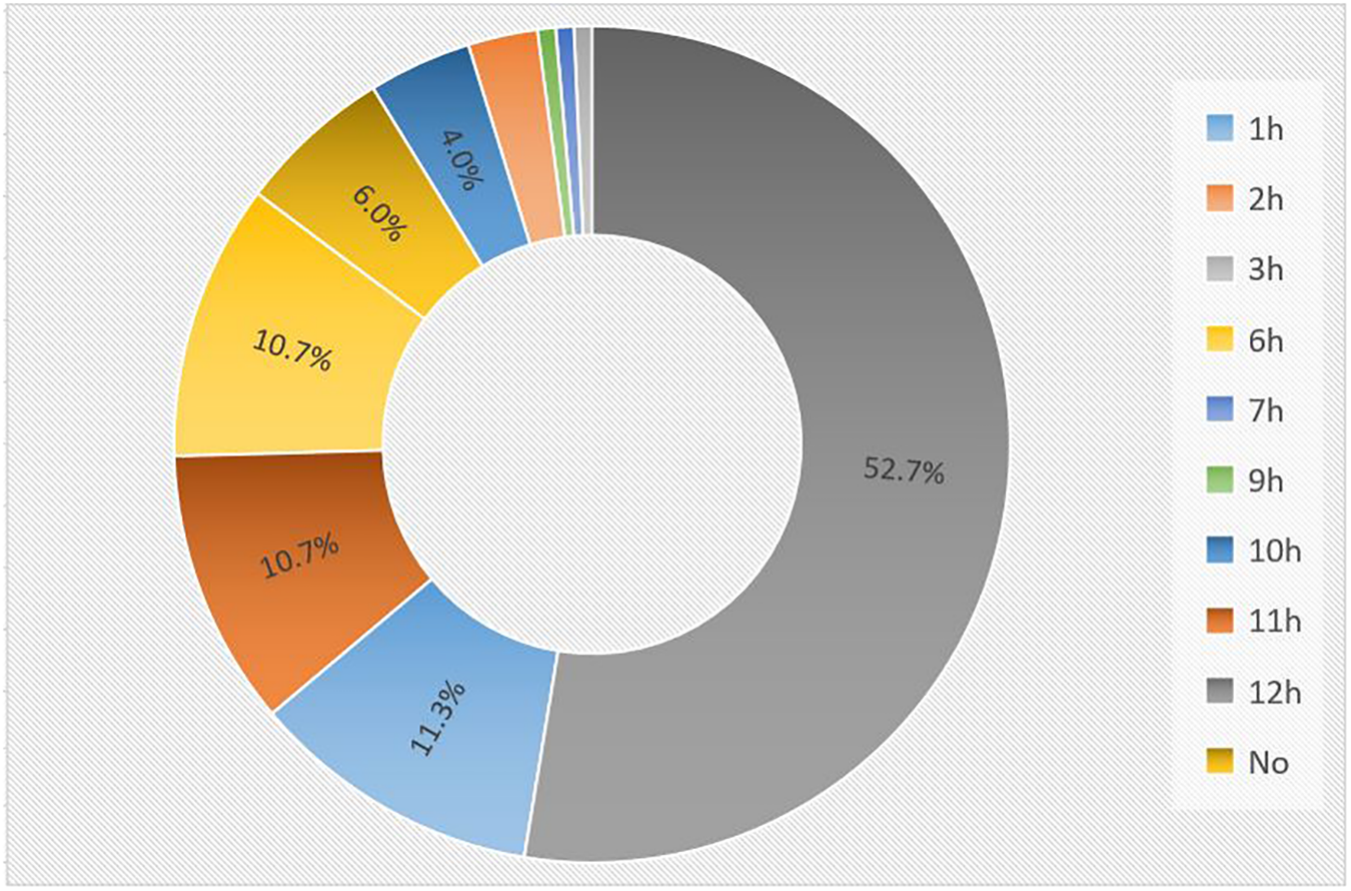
The distribution of internal openings in MR imaging. The most common location of the internal openings was from 11 o'clock to 1 o'clock (74.7%), followed by 6 o'clock (10.7%). The remaining positions were less common.
